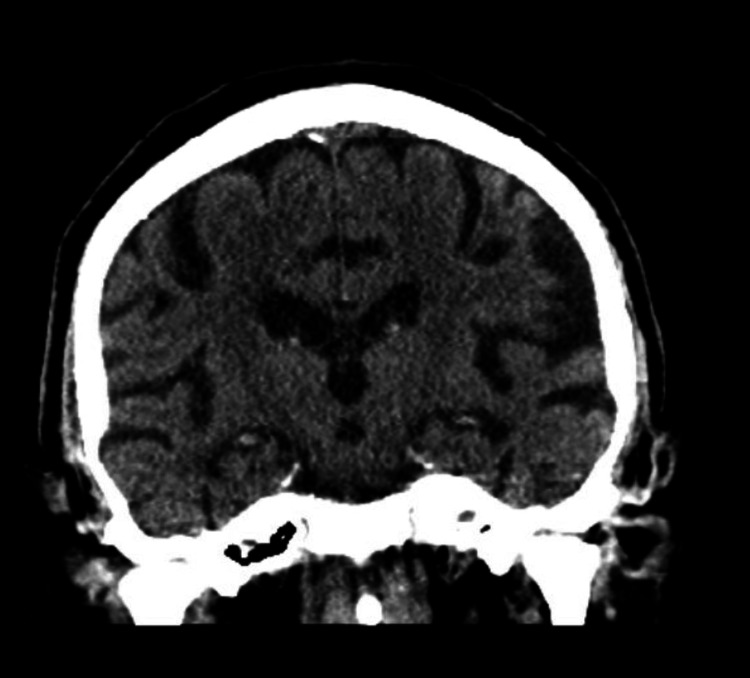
CT scan head without contrast Generalized cerebral volume loss
